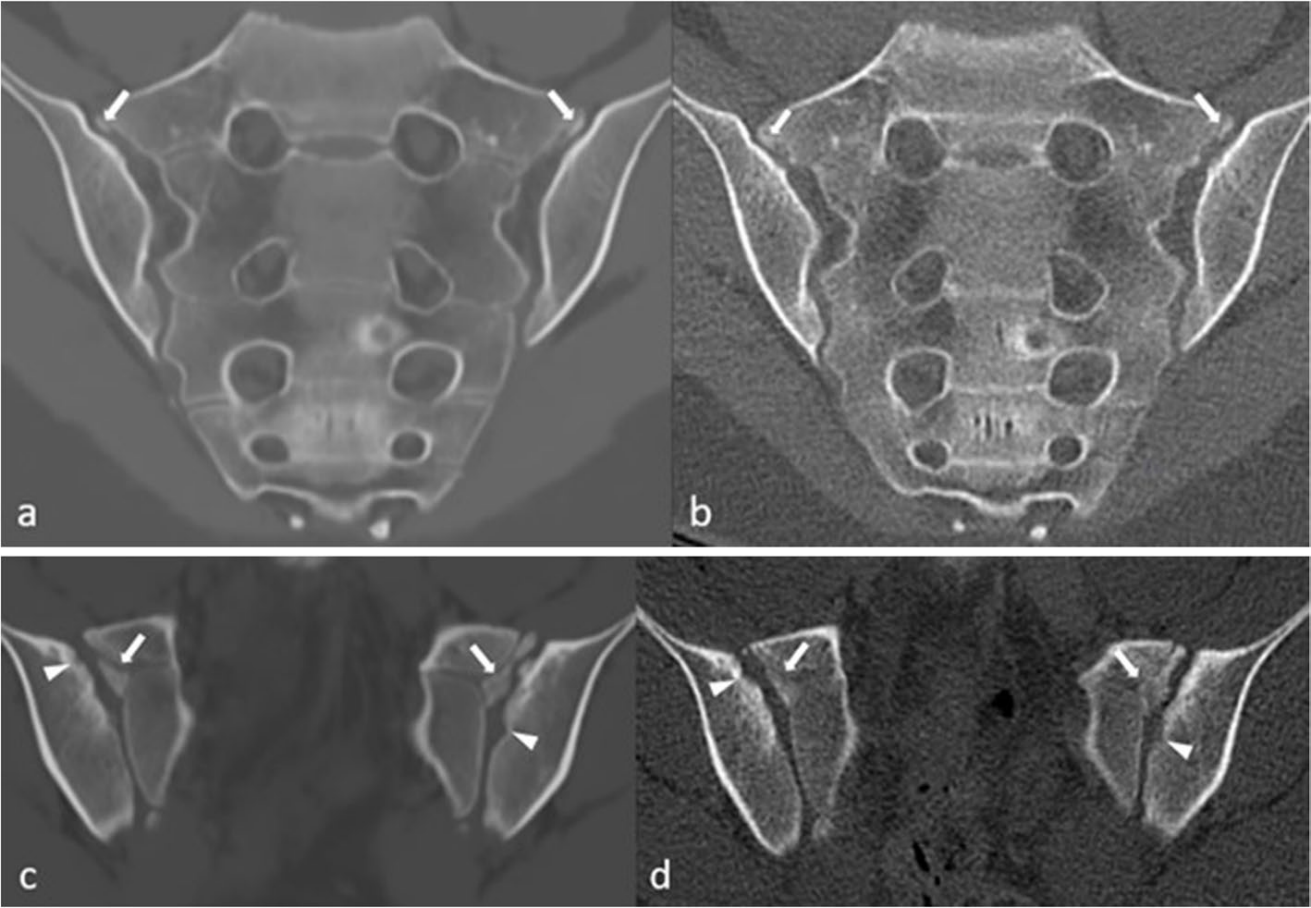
Comparison between MRI-based synthetic CT (sCT) (a, c) and CT (b, d) in a 15-year-old girl in a paracoronal plane. Arrows indicate ossified nuclei. Also note the irregularities seen on sCT and CT (arrowheads) on the iliac side of the sacroiliac (SI) joints, these did not meet the criterion of ≥ 3 mm for joint facet defect
